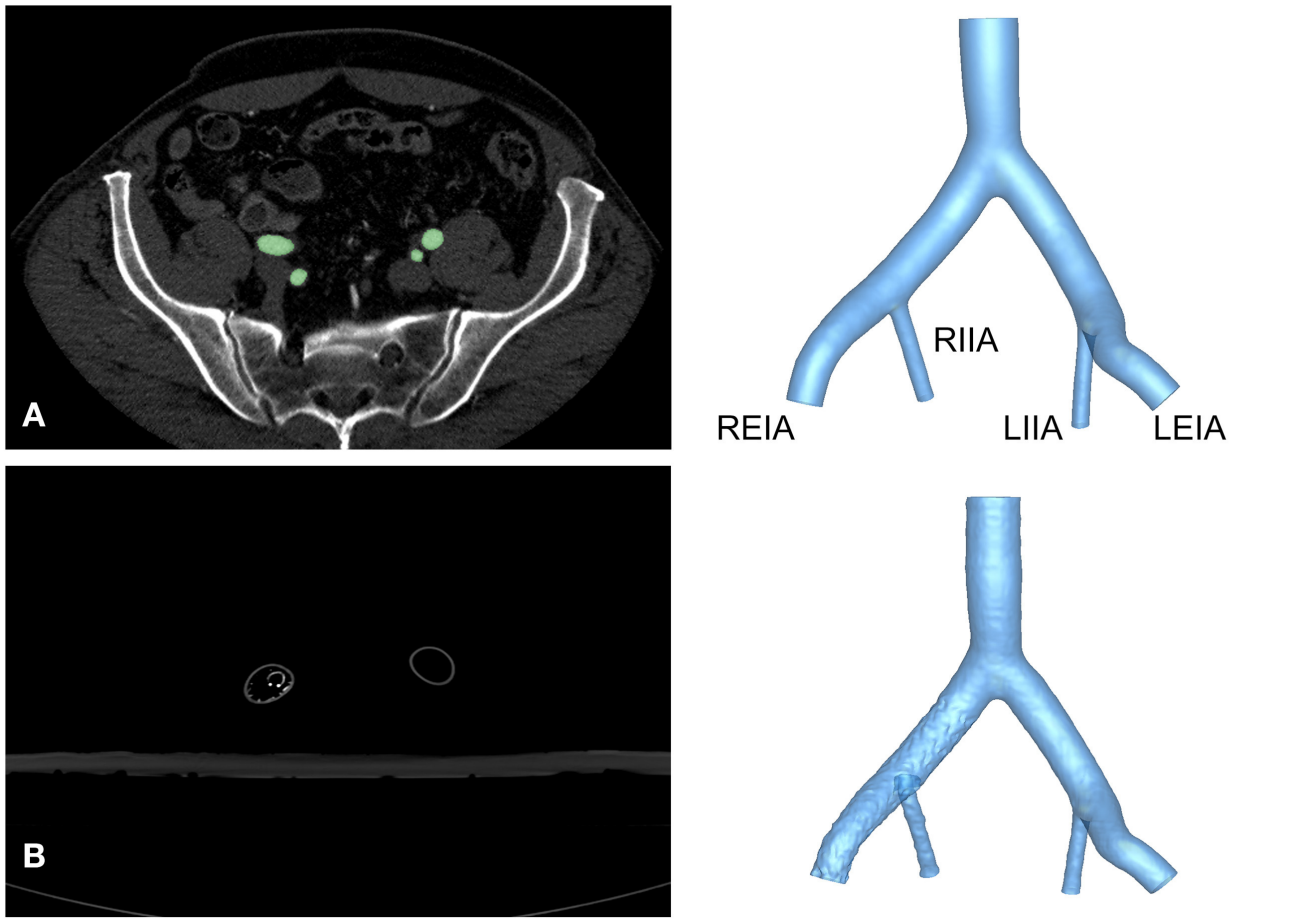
Reconstruction of the iliac artery model. (A) Axial view of CTA images from a healthy volunteer and reconstructed structure of Model A. (B) Axial view of CTA images and reconstruction of Model B. LEIA, left external iliac artery; LIIA, left internal iliac artery; REIA, right external iliac artery; RIIA, right internal iliac artery.
